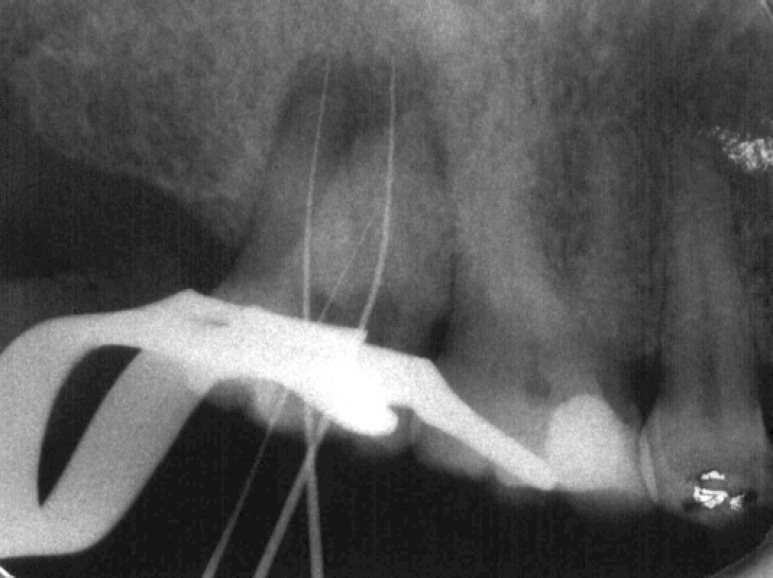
Working length determination X-ray
